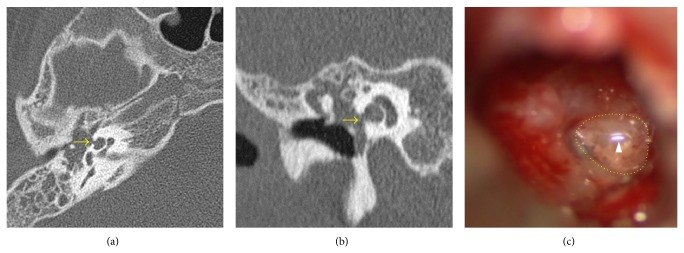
(a) Axial and (b) coronal CT scans of right temporal bone showing cholesteatoma with cochlear fistula (arrow). (c) Intraoperative findings confirmed the fistula with pulsatile fluid. The arrow head indicates light reflex in the fluid.
